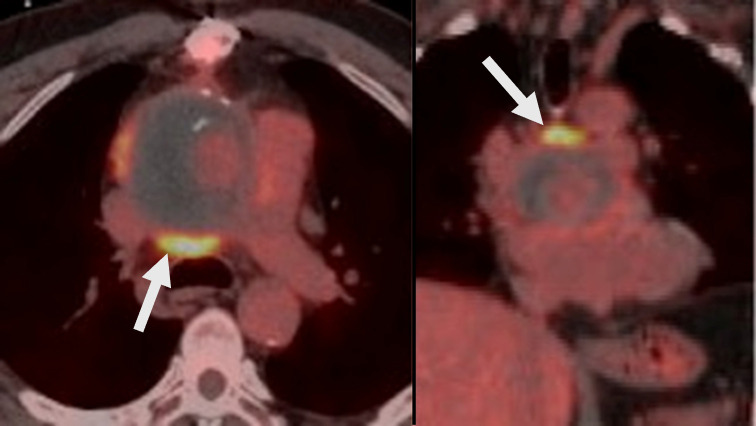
Pseudoaneurysm PET/CT. F-18 FDG PET/CT axial (left) and coronal (right) views. Depicts postoperative imaging following aortic root repair with fluid collection surrounding the aortic root. Along the periphery of this collection, there is avid FDG uptake (arrow) with SUV maximum up to 10.5, potentially inflammatory, though highly suspicious for infection given the degree of FDG uptake.
